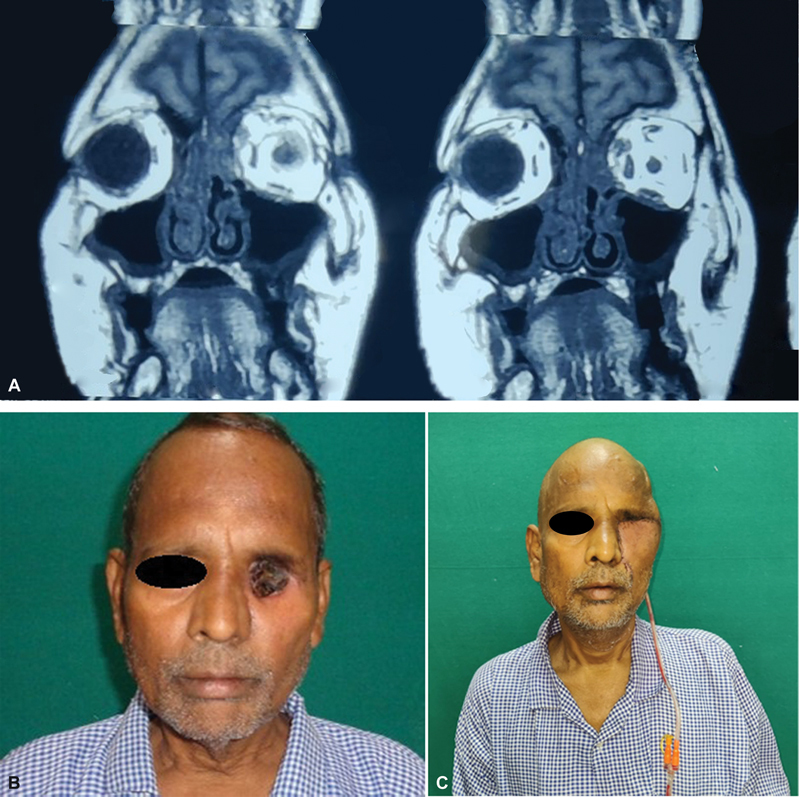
(A) Magnetic resonance imaging showing left orbital involvement. (B) Postdebridement defect. (C) Reconstruction with temporalis muscle and cheek advancement flap.
